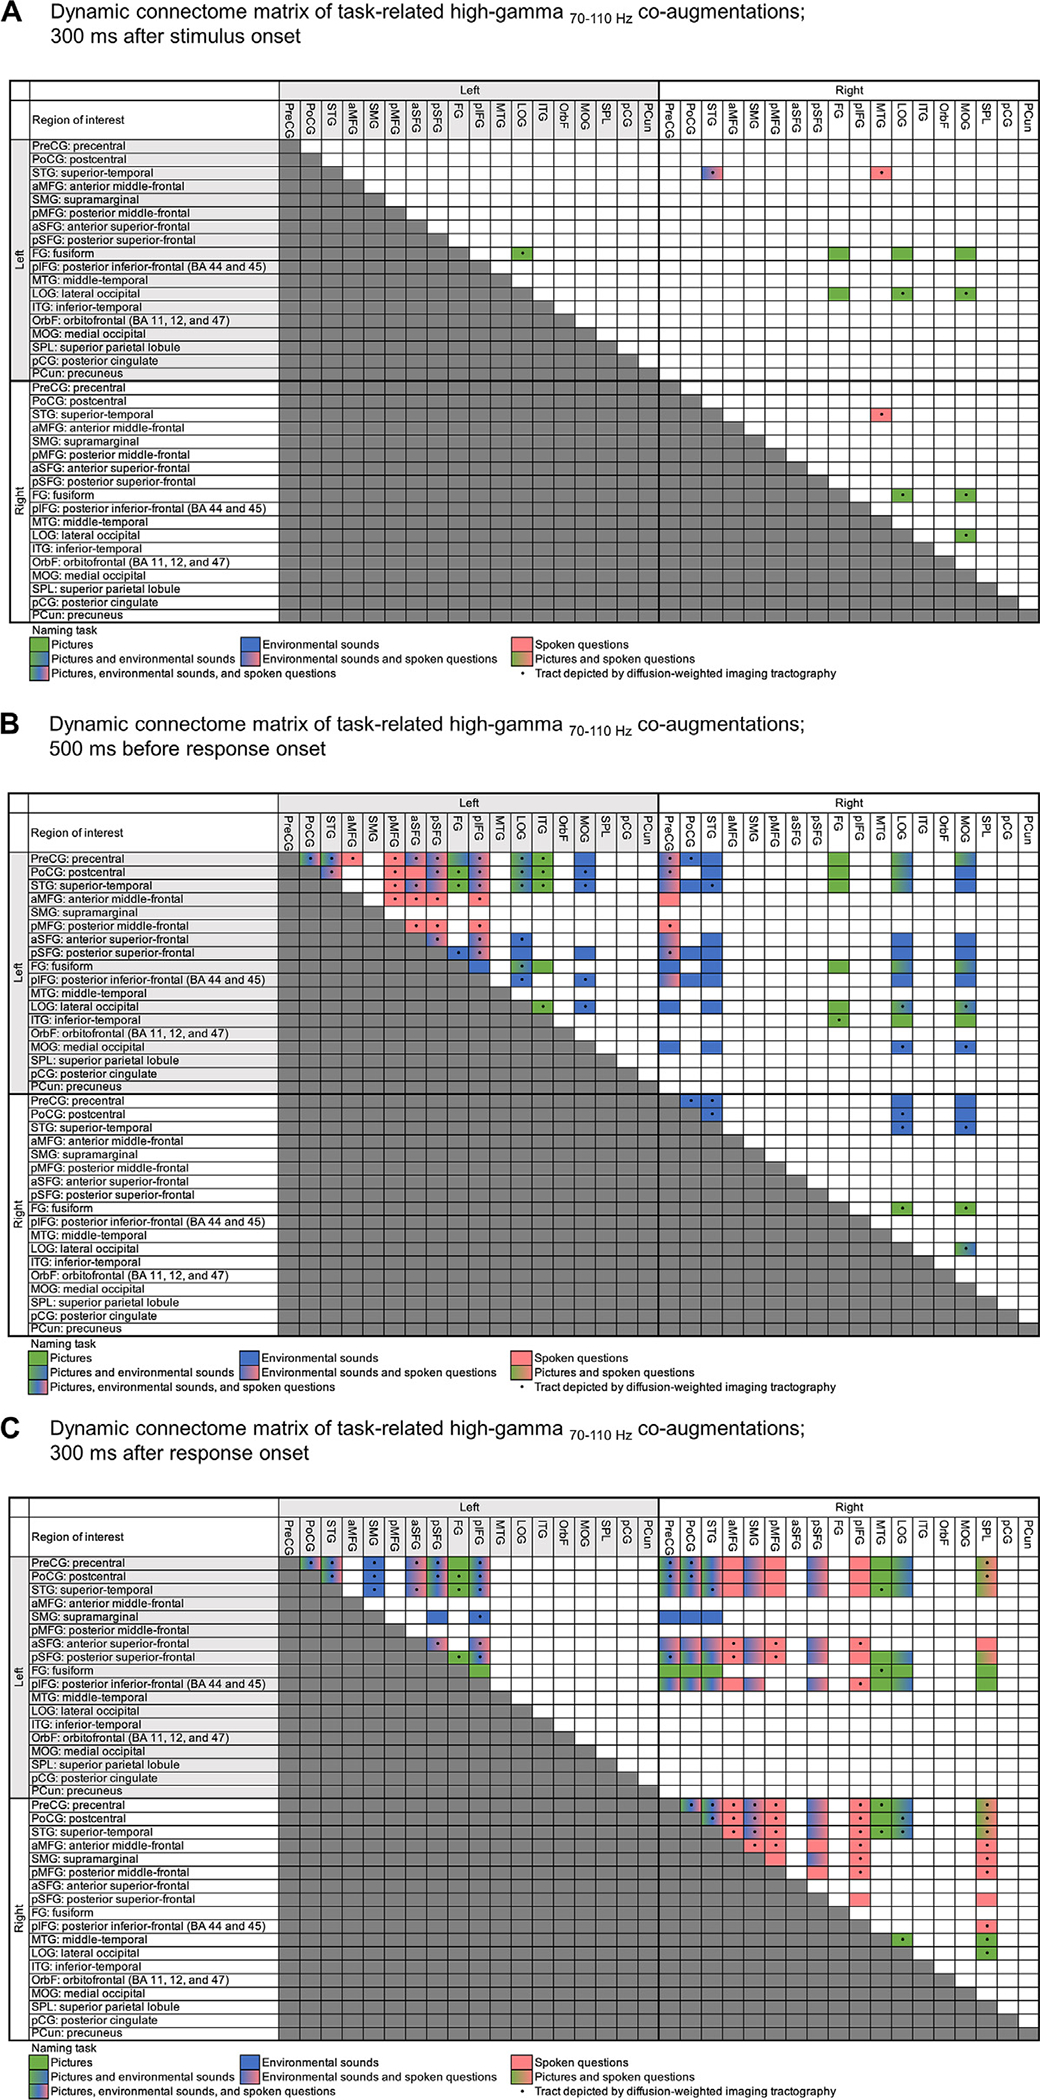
Snapshots of dynamic connectome matrix. The color of each box indicates the given naming task(s) that elicited significant direct functional connectivity enhancement between the two corresponding regions of interest (ROIs) at (A) 300 ms after stimulus onset. (B) 500 ms before response onset. (C) 300 ms after response onset. Functional connectivity was considered enhanced when two ROIs linked via direct DWI streamlines showed significant (based on the studentized bootstrap statistics), simultaneous, and sustained (≥100 ms) high-gamma co-augmentation. Light green: picture naming. Blue: non-speech environmental sound naming. Magenta: auditory descriptive naming. Video S5 shows the dynamic connectome matrix as a function of time.
